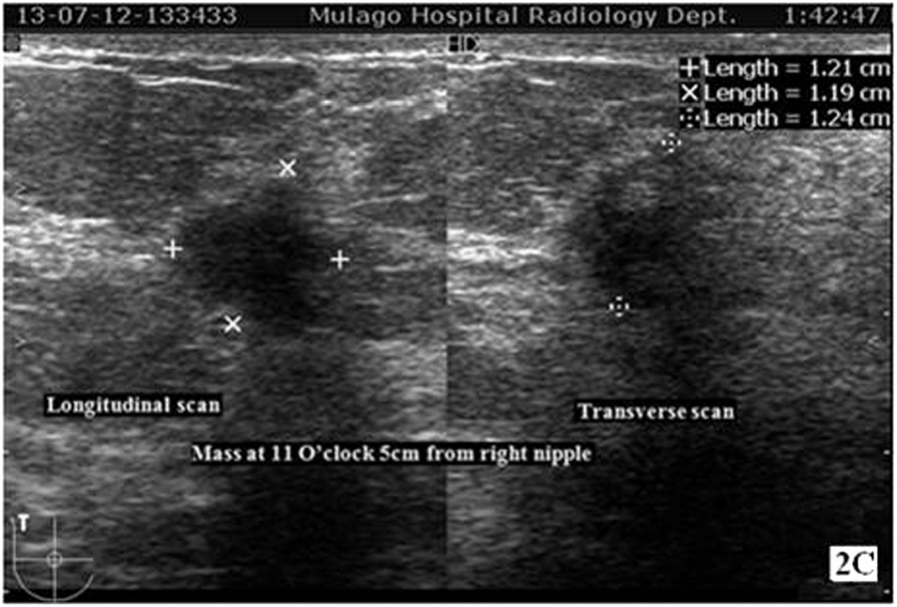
Sonogram shows a solid hypoechoic mass which is irregular in shape, has angular margin with surrounding echogenic halo at 11 O’clock 5 cm from right nipple.
